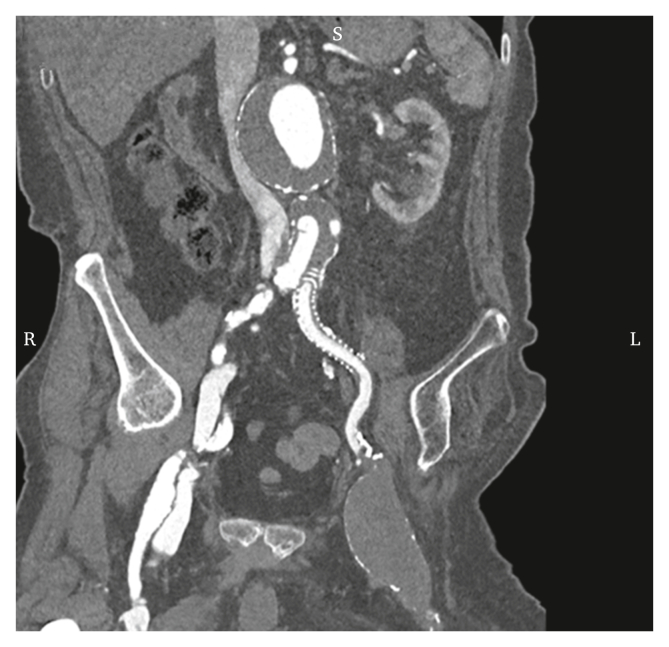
Coronal multiplanar reconstruction of the post-operative computed tomography angiogram, demonstrating successful ligation of the external iliac artery and exclusion of the common femoral artery aneurysm.
